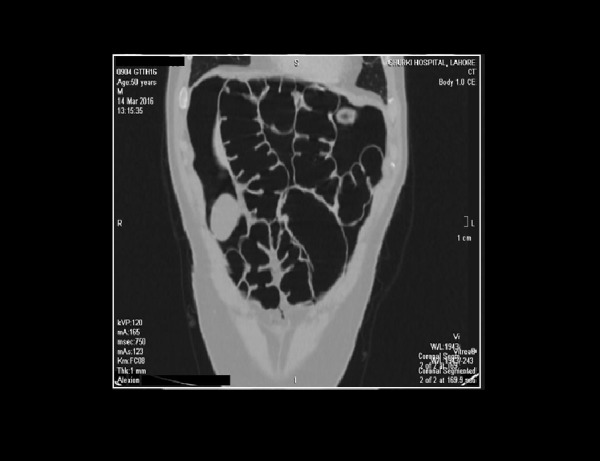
A coronal section of the CT abdomen showing pneumo-peritoneum along with pneumatosis intestinalis and thick reactive intestine walls. The radiologic presentation assures the presence of air in the gut, which can be due to a perforation.
